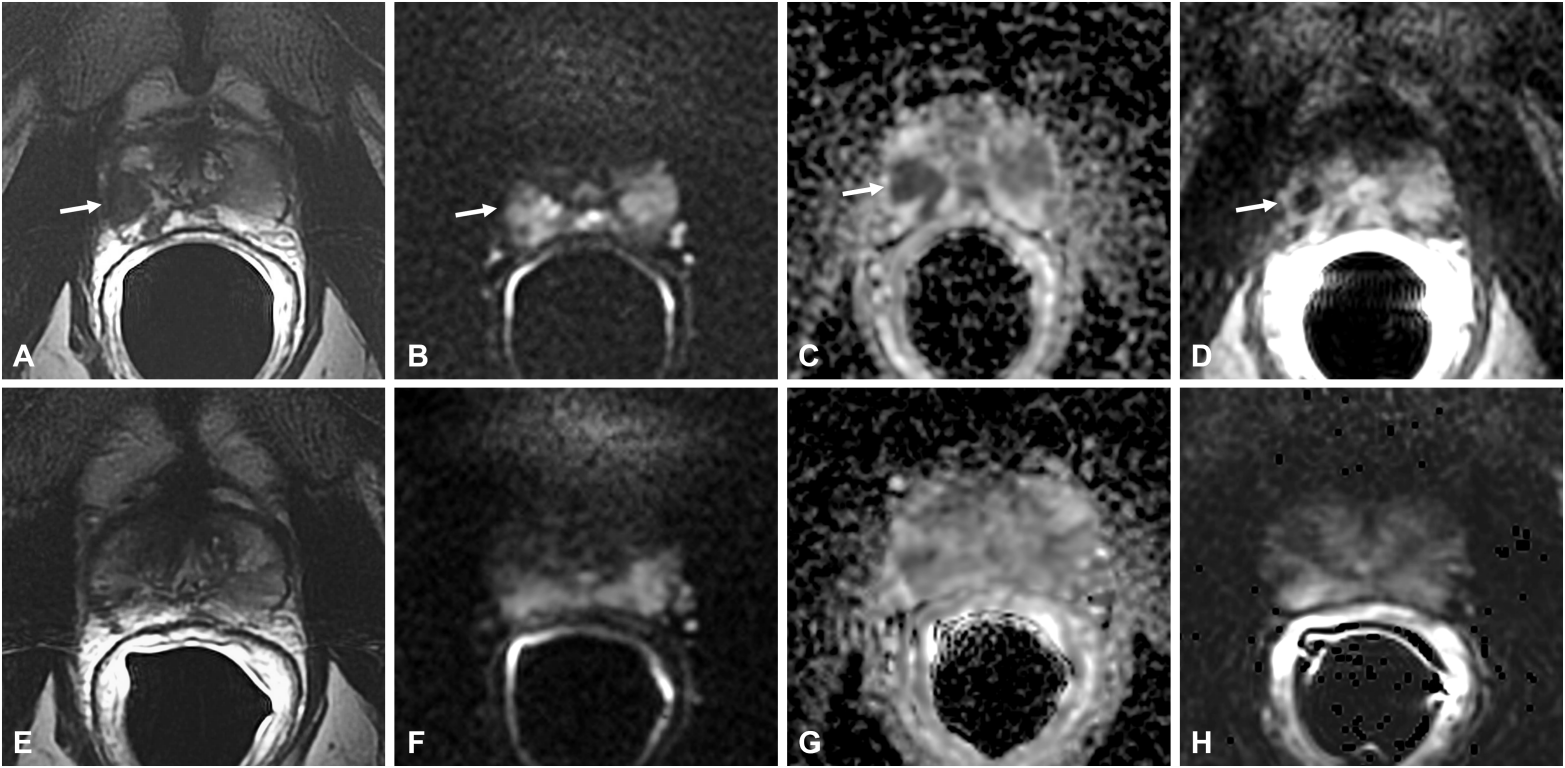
Bacillus Calmette-Guérin-induced granulomatous prostatitis in a 58-year-old patient with a PSA value of 1.17 ng/ml. The first mpMRI at diagnosis showed diffuse alteration of the peripheral zone (PZ) with a hypointense nodule in the right middle lobe in the T2-weighted sequence (arrow in A) with hyperintensity on the DWI image (arrow in B), marked hypointensity on the ADC map (arrow in C), and typical “ring enhancement” on the DCE image (arrow in D). The corresponding sequences of the 5-month mpMRI follow-up (E–H) showed almost complete resolution of the aforementioned findings.
